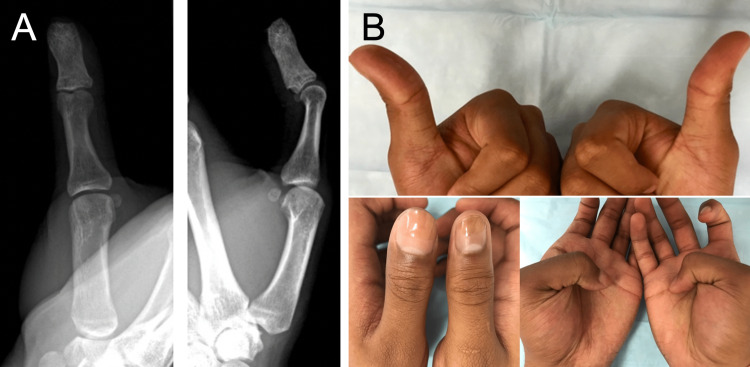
X-rays and clinical pictures of the left thumb seven months postoperatively X-ray images (A) and clinical pictures (B) are showing the formation of the distal phalanx in an appropriate position in relation to the proximal phalanx with an intact range of motion.
